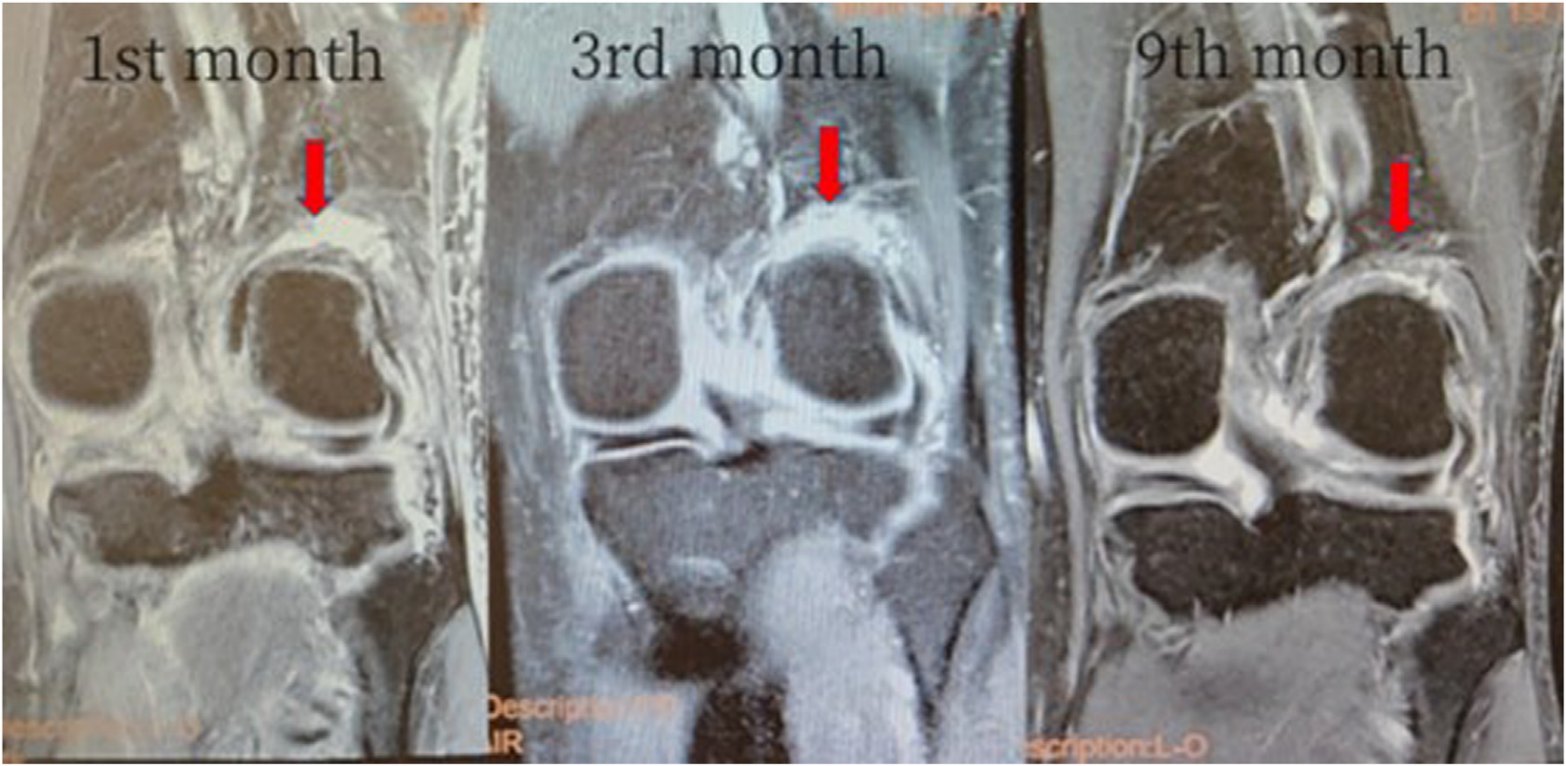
Serial coronal T2-weighted MRI of a 35-year-old male patient after left knee OTT + anterior lateral ligament combined reconstruction surgery acquired 1, 3, and 9 months postoperatively. The arrow shows that the boundary between the transplanted tendon in the OTT part and the surrounding tissues has become gradually blurred. (MRI, magnetic resonance imaging; OTT, over-the-top.)
